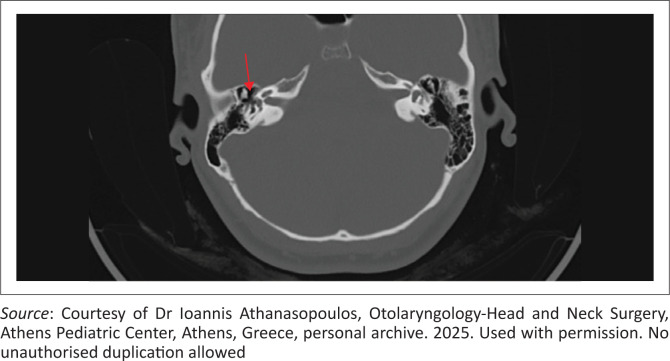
Axial CT image of the temporal bone in a 24-year-old female patient with otosclerosis, showing a hypodense focus in the otic capsule anterior to the oval window (fissula ante fenestram, red arrow), characteristic of fenestral otosclerosis. This lesion leads to conductive hearing loss by fixation of the stapes footplate.
